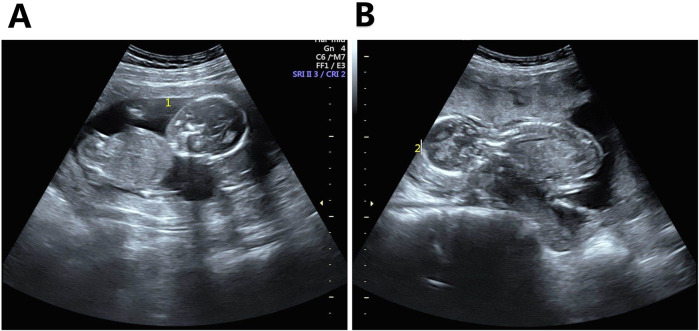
Ultrasound images of the two fetuses before fetal reduction. Note: (A) Amniotic cavity of Fetus 1 showing almost no amniotic fluid; (B) Normal amniotic fluid volume in Fetus 2.
